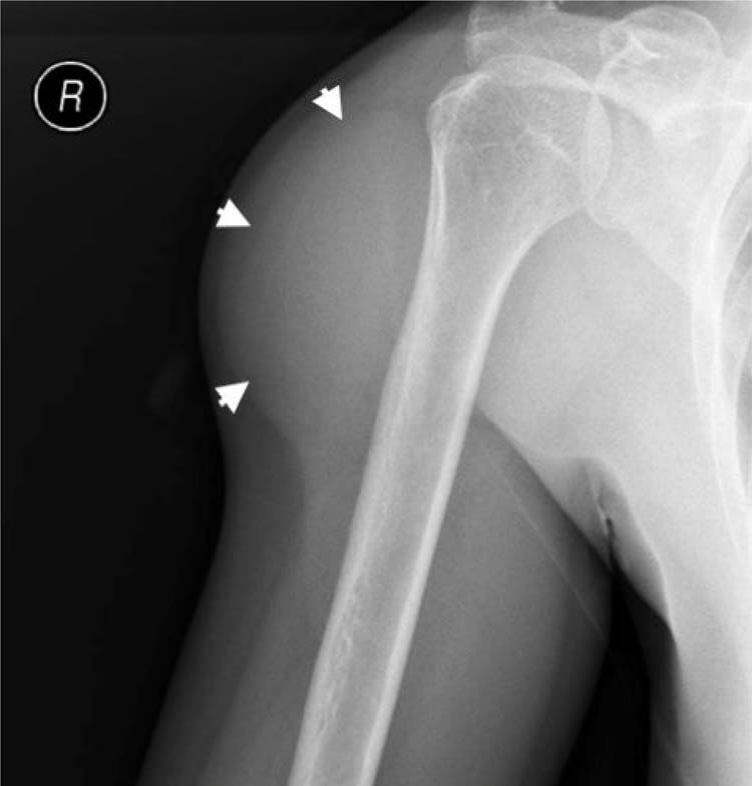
Externally rotated AP radiograph of the right shoulder and upper-arm. Short arrows indicate the tumoral mass.
